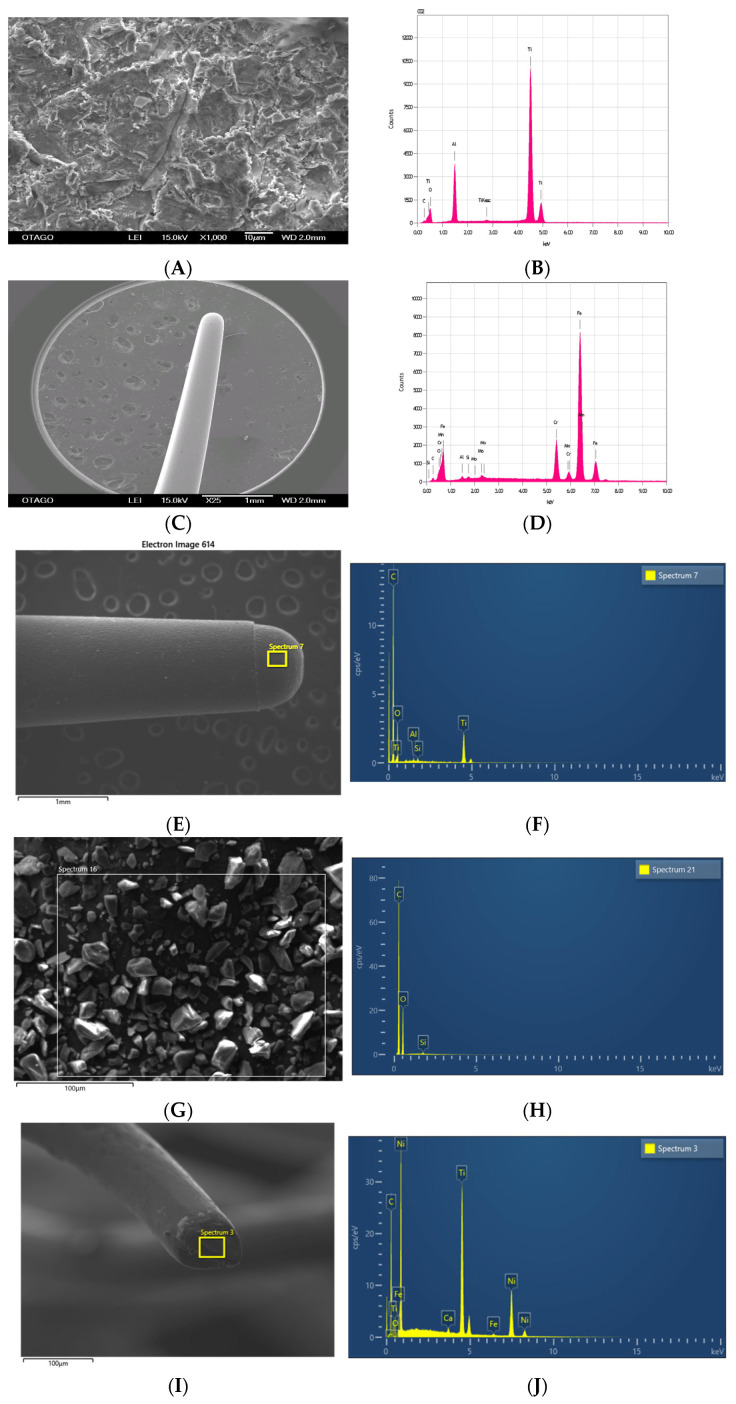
Chemical composition of Ti disc and instruments under ×1000 magnification: moderately roughened Ti disc (A,B), ultrasonic metal tip (C,D), ultrasonic PEEK tip (E,F), erythritol powder (G,H), and Ti brush (I,J).
